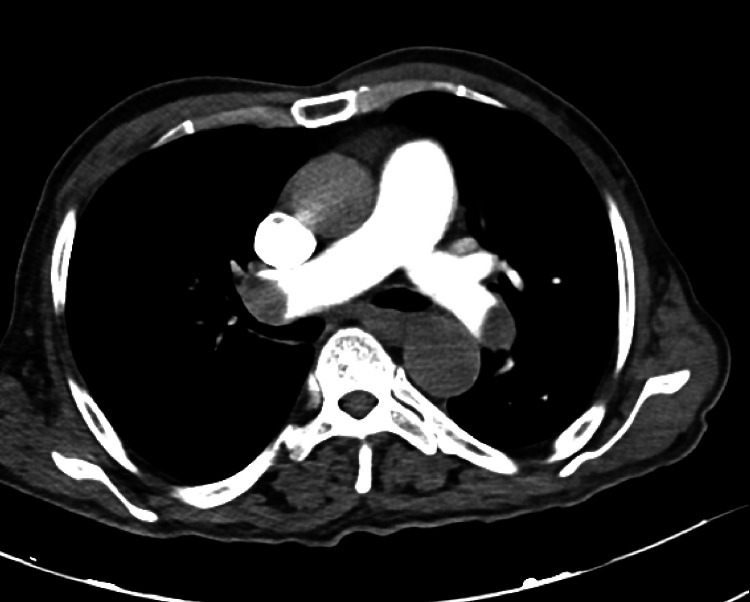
CT pulmonary angiography revealing thrombus
